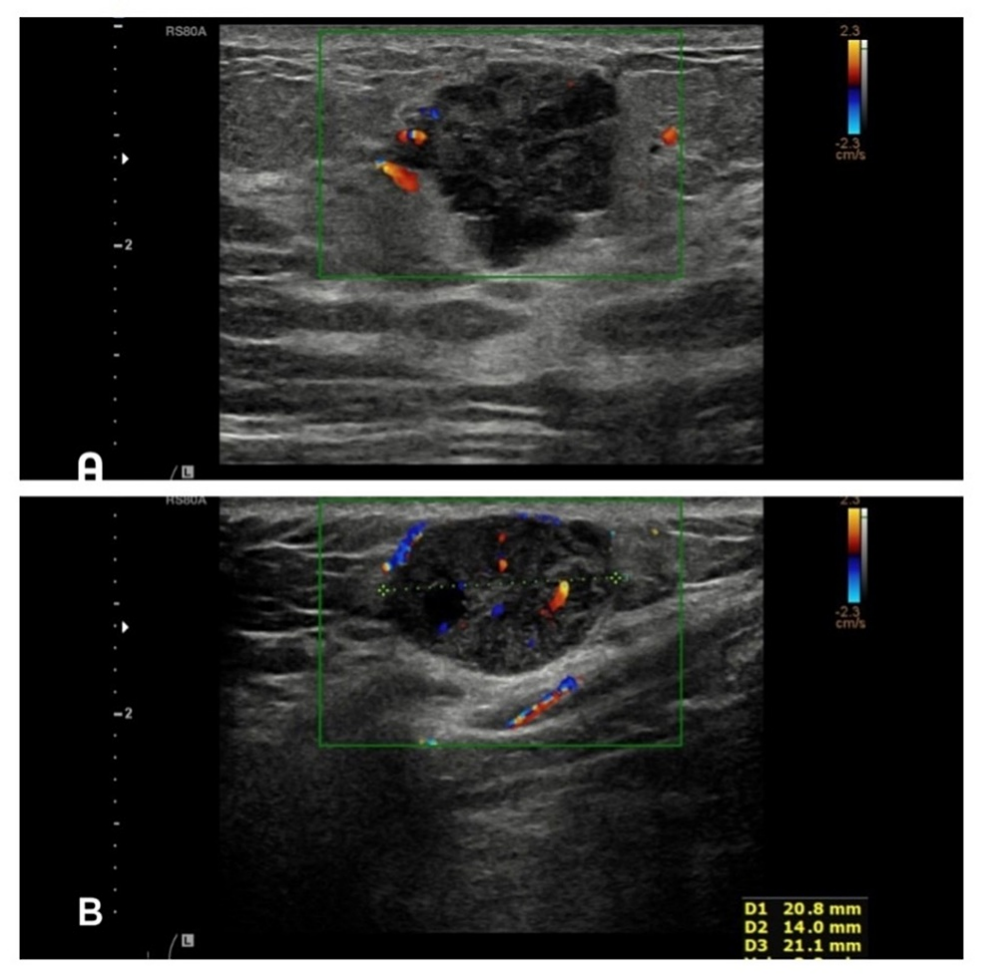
A - Ultrasound left breast revealed an indeterminate lesion measuring 22 mm at 10 o’clock position with solid components and vascularity. B - Ultrasound subcutaneous lesion in the left inframammary fold: indeterminate lesion measuring 21 mm.
